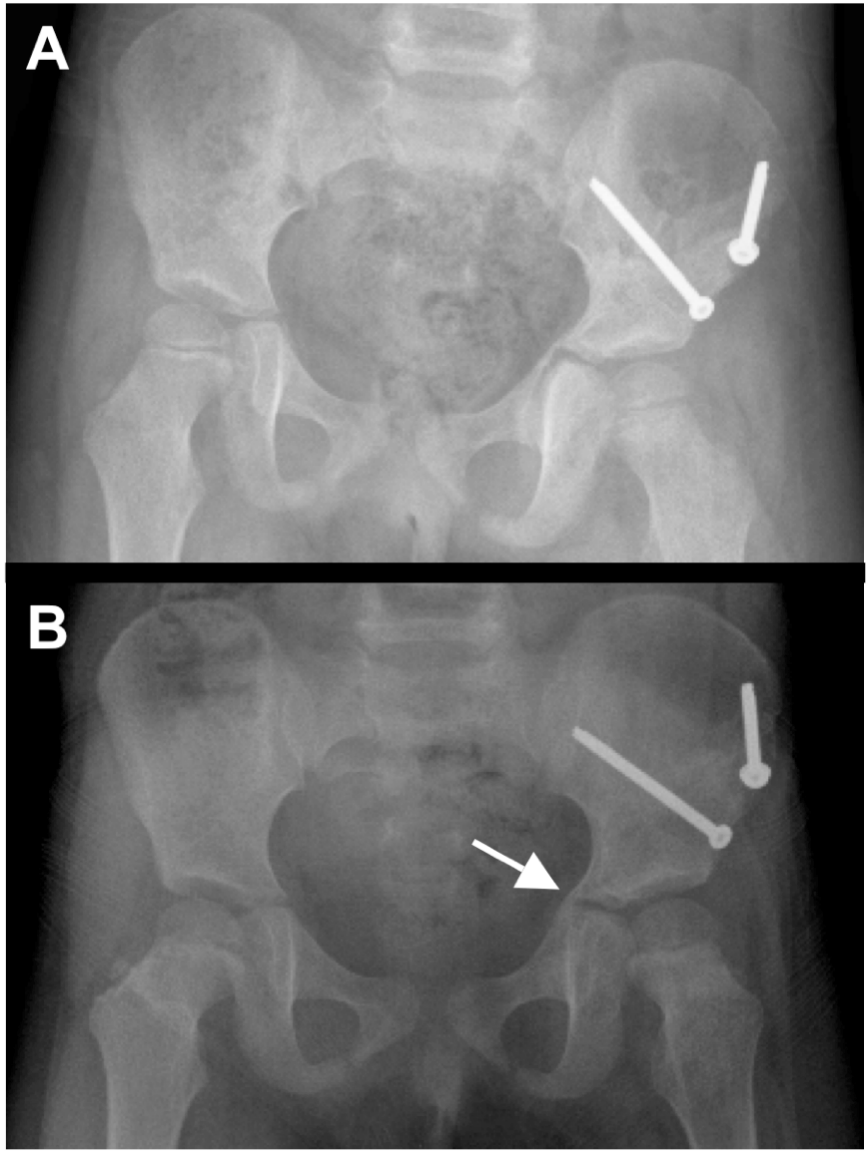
Follow-up X-rays taken at 8 weeks (A) and 4 months (B) demonstrate a healed left acetabular fracture in anatomic position and indicate an early physeal closure with callus formation about the tri-radiate cartilage (arrow in panel B).
